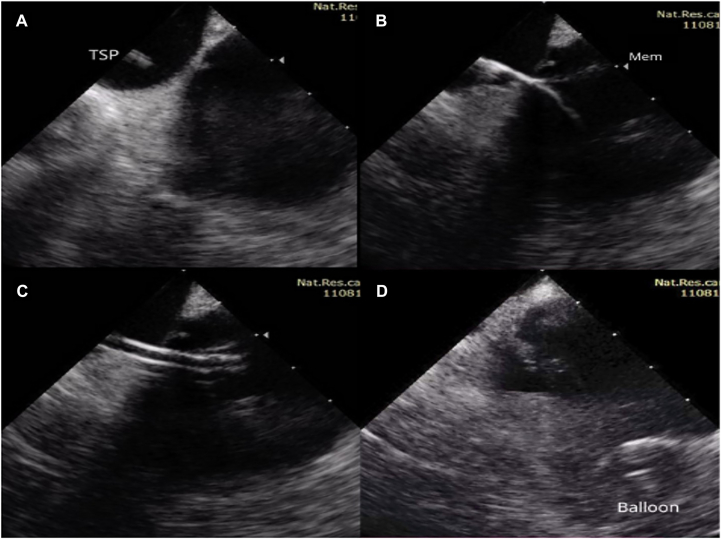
Two-dimensional intracardiac ultrasound modeling images. A: The transeptal puncture needle is positioned at the posterior interatrial septum. B: Transeptal puncture and guidewire introduction into LA. C: The FlexCath Steerable Sheath was introduced to the LA. D: Cryoballoon ablation of the left superior pulmonary vein. Balloon = inflated cryoballoon; LA = left atrium; Mem = fibromuscular membrane; TSP = transeptal puncture needle.
